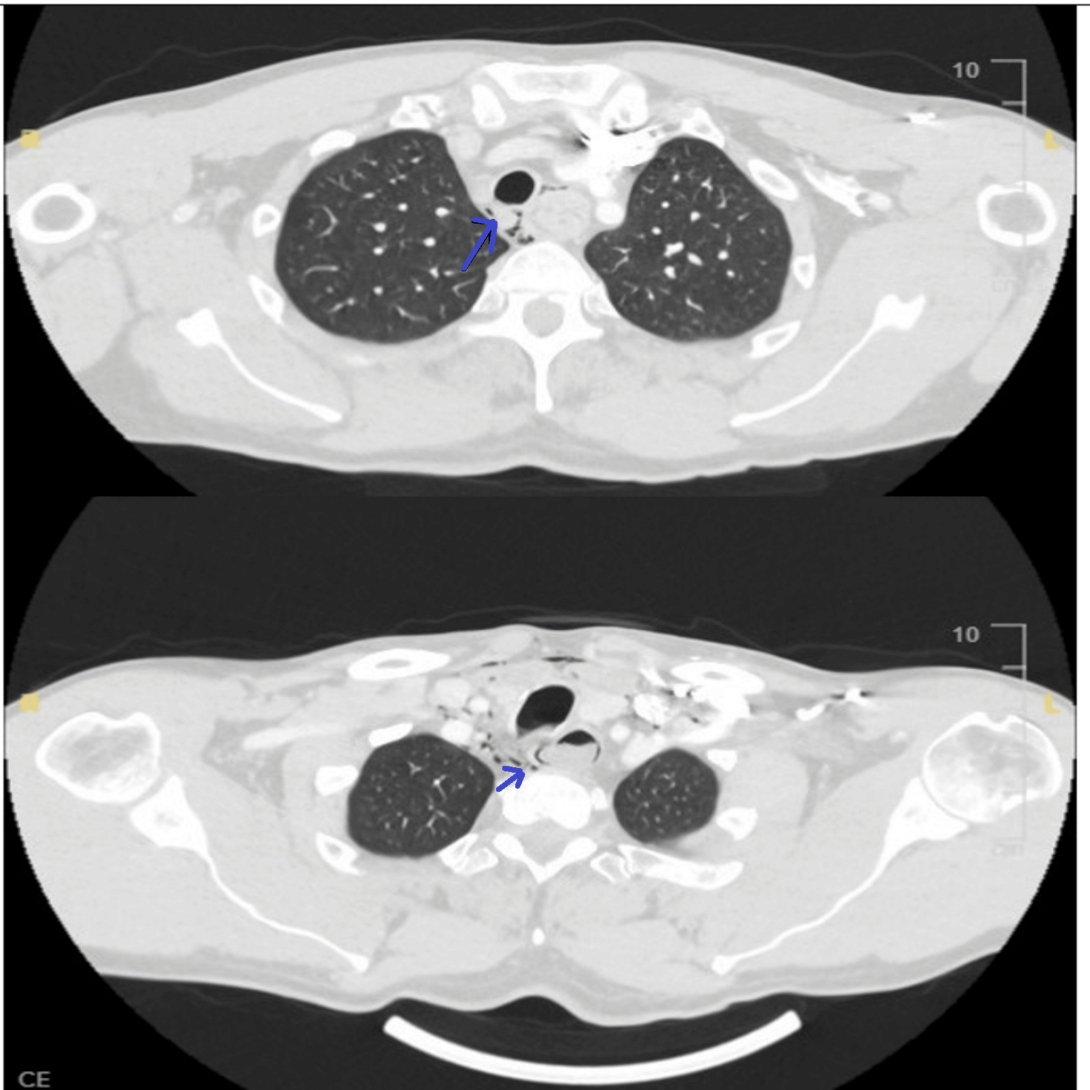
CT of the neck and thorax with contrast Pneumomediastinum noted mainly posteriorly along with soft tissue neck emphysema. Appearances are compatible with esophageal rupture.
